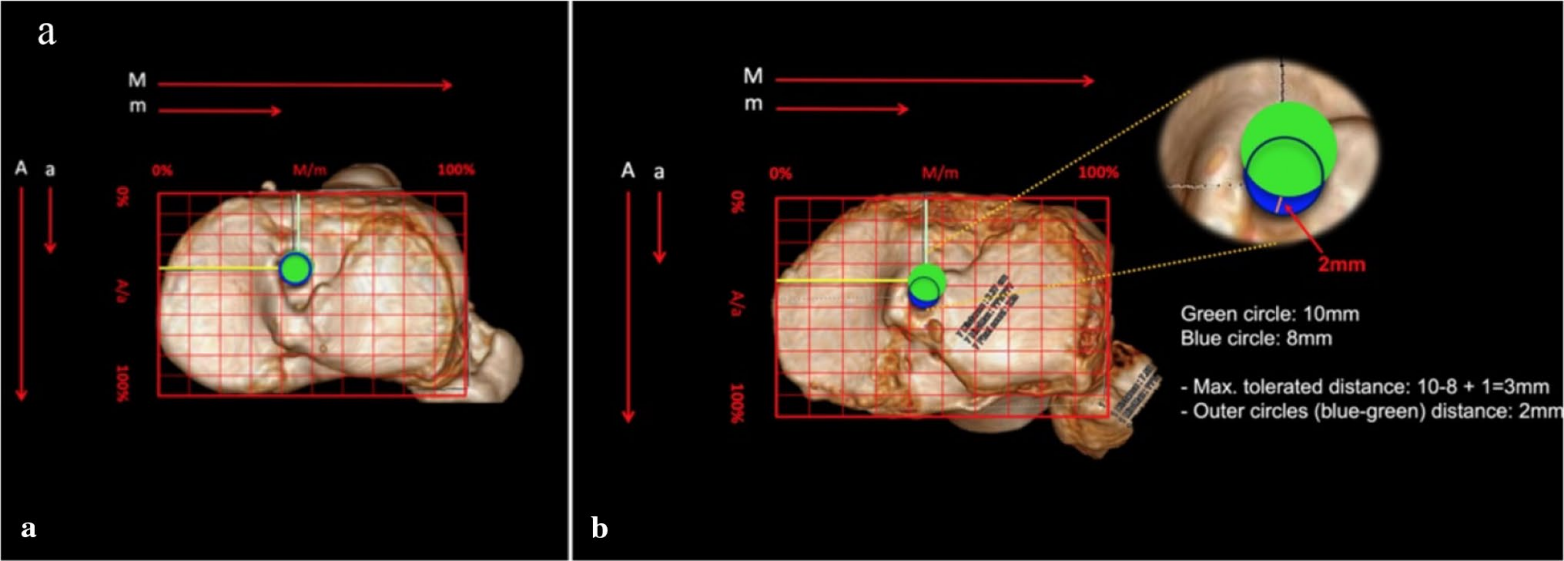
A 3D-CT reconstruction of the tibial plateau in the axial view with complete superimposition between the previous (blu) and the new planned tunnels (green); B 3D-CT reconstruction of the tibial plateau in the axial view with tolerated superimposition, candidate for single-stage revACLR (colour figure online)
